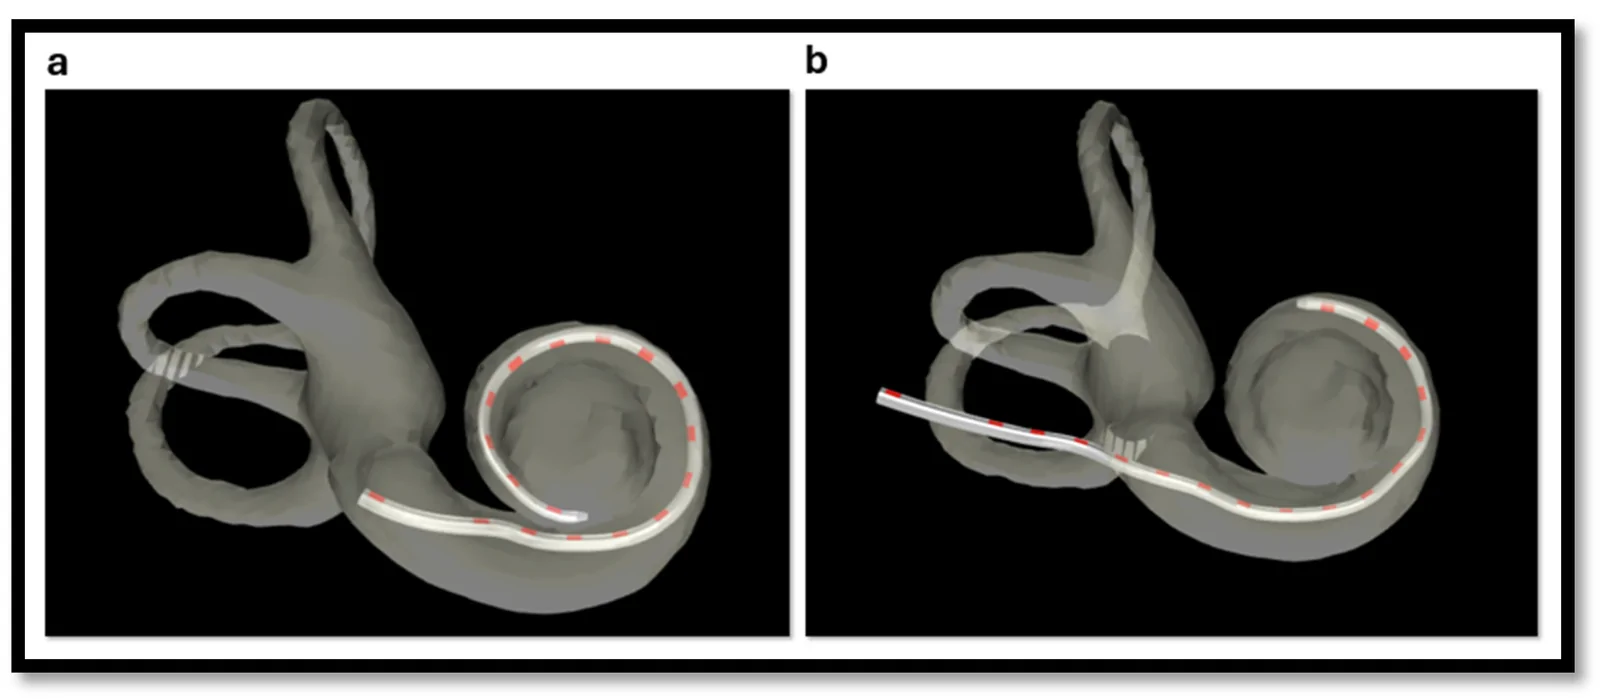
Cochlear implant electrode localization from postoperative CT scans generated using Vanderbilt University’s Oto-pilot system. (a) Example of full intracochlear insertion. (b) Example of a case with three EEs identified from CT reconstruction.
